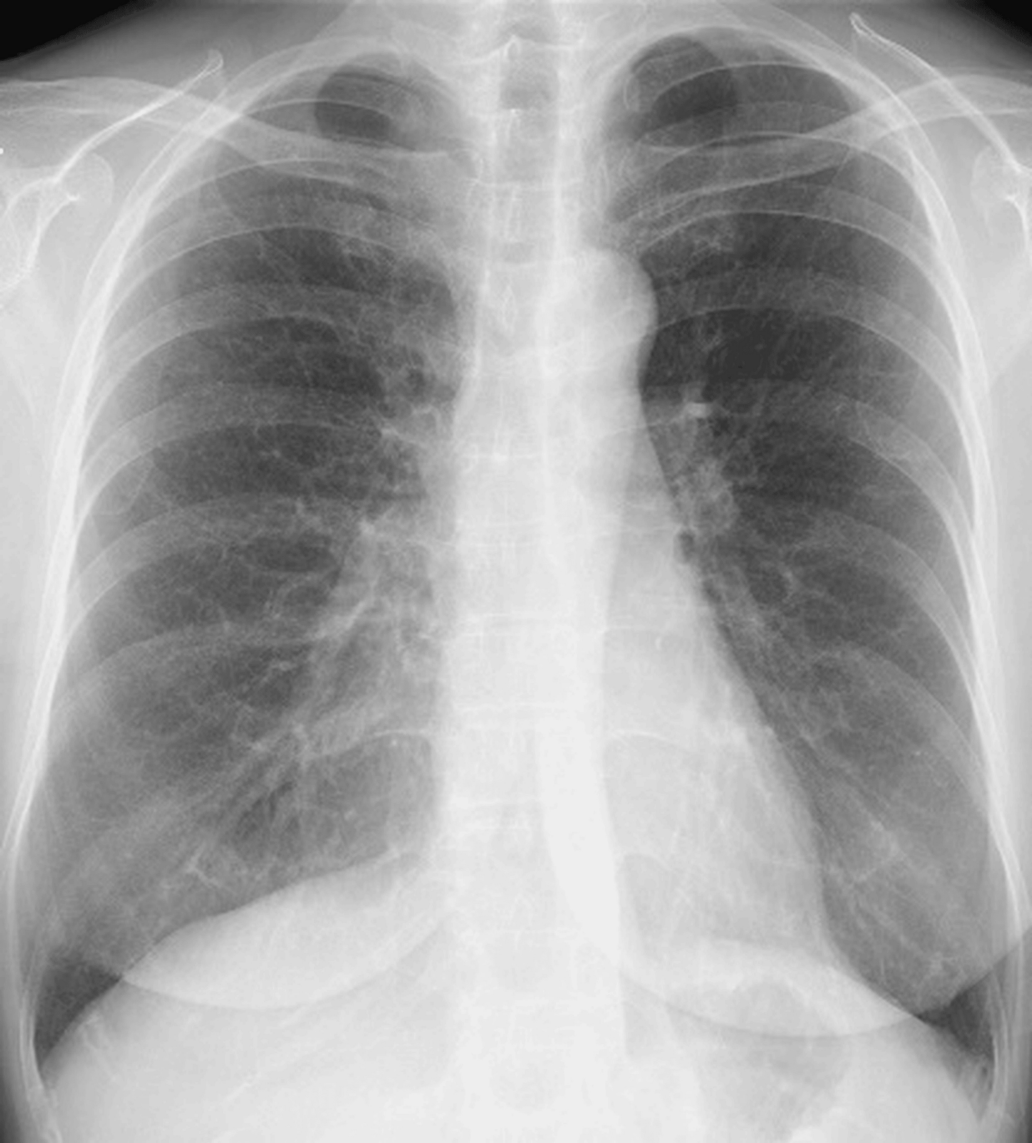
Chest radiograph on admission Chest radiograph showing bilateral fine granular opacities.
